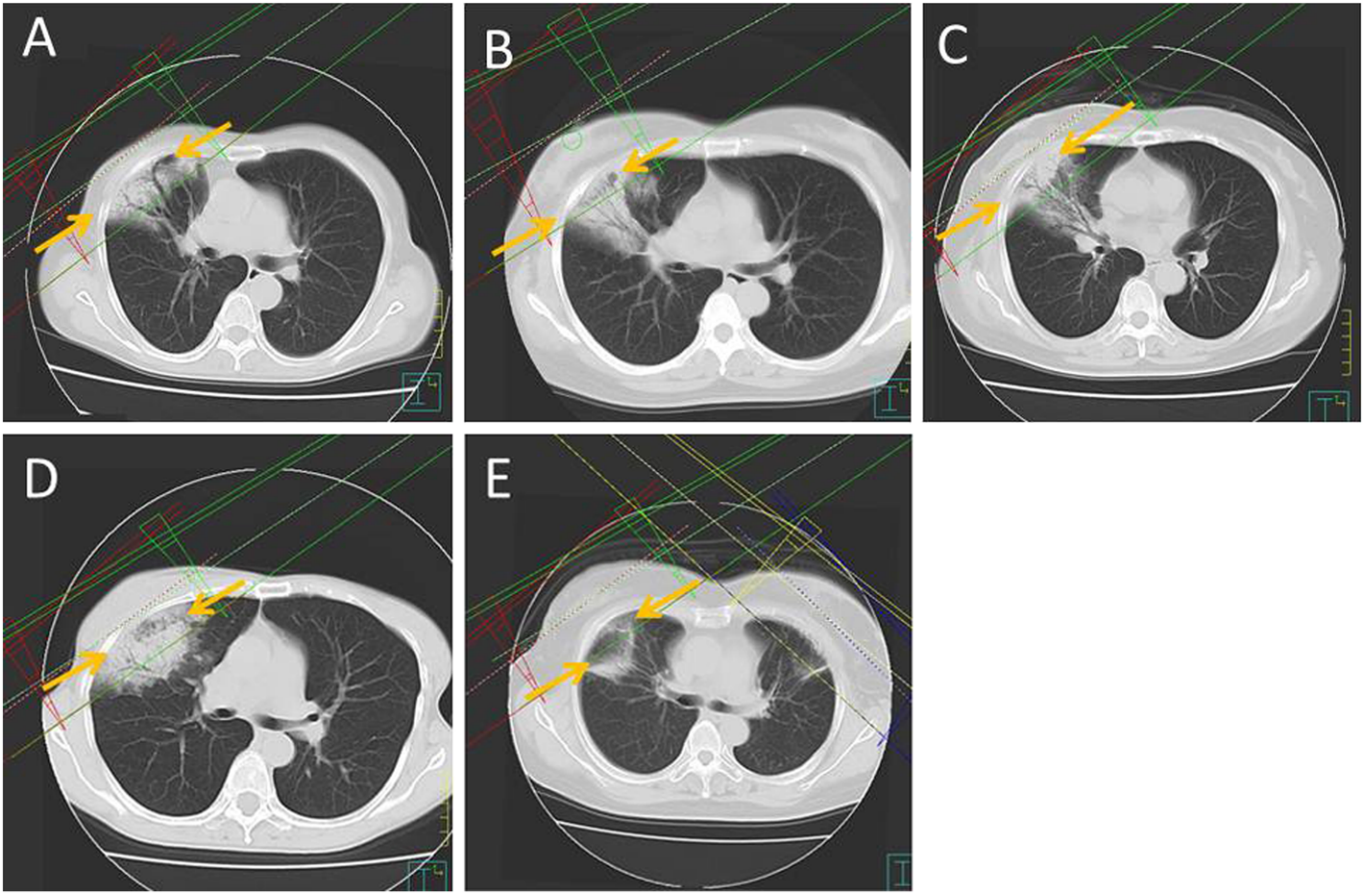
Fusion of CT images at the time of diagnosis of OP and treatment planning images (A = patient 1, B = patient 2, C = patient 3, D = patient 4, E = patient 5). There were free regions between the radiation lesions and the radiation-induced OP (arrow).
